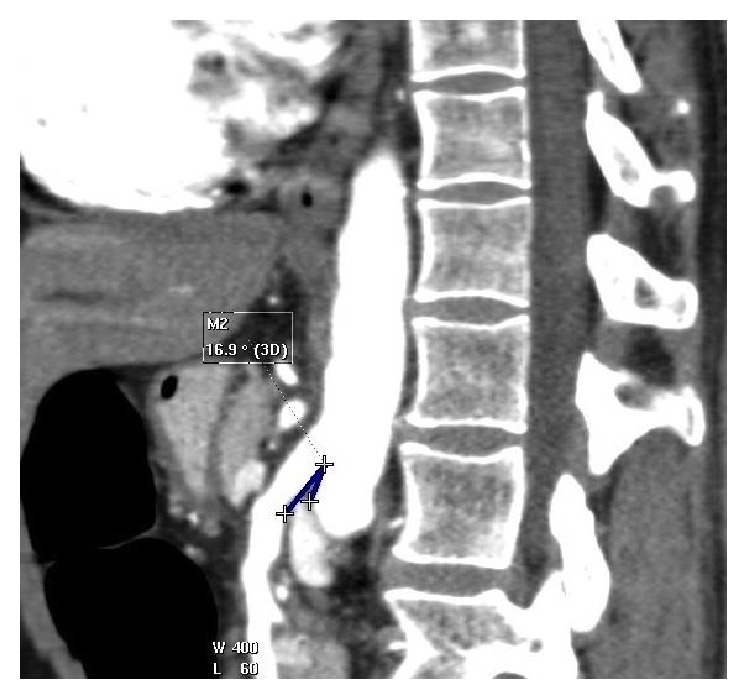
CT sagittal view showing angle of 16.9 degrees between the superior mesenteric artery and aorta (Case Report One).
